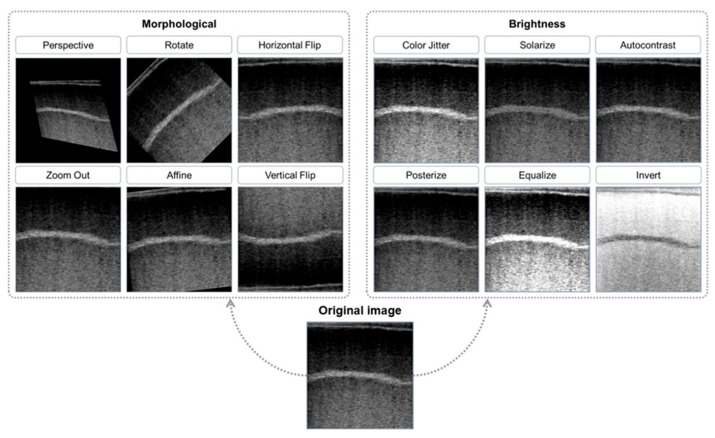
Image augmentation techniques applied to testicular ultrasound images, including morphological transformations (e.g., perspective, rotation, flipping) and brightness adjustments (e.g., color jitter, solarization, autocontrast). These augmentations were used to assess their impact on model performance in predicting semen quality.
